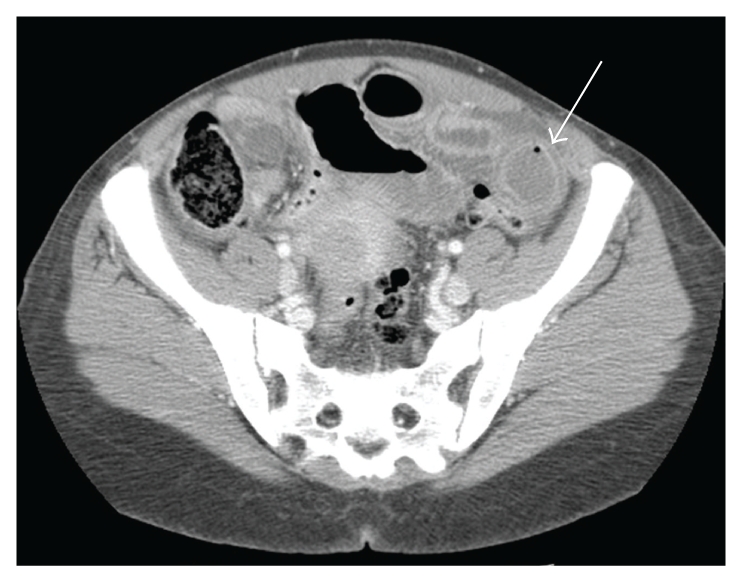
CT at the time of symptom recurrence exhibiting dilated small bowel with mural stratification.
